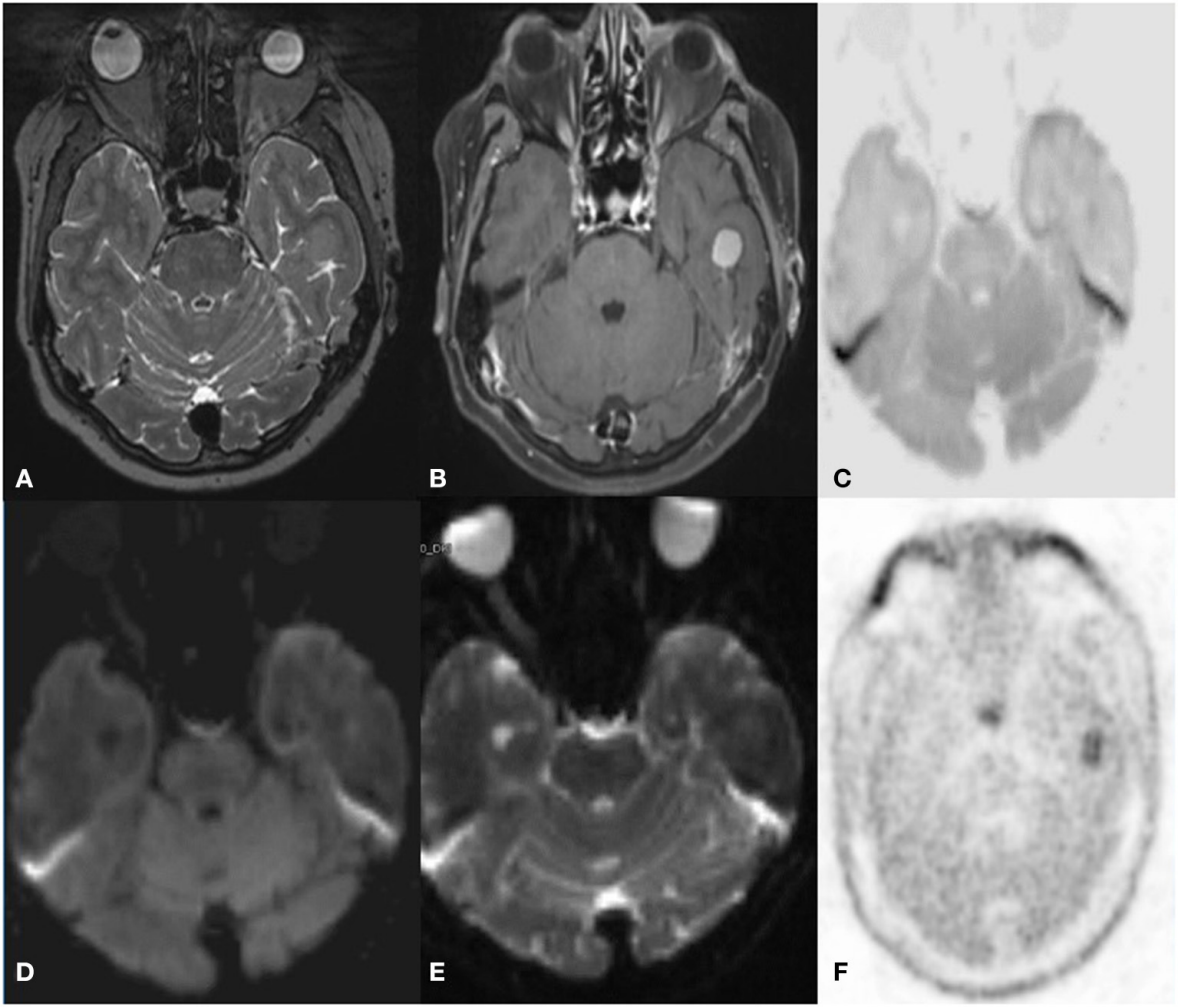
A 59 year old female with Glioma, incidentally detected a lesion in follow up MRI C-11 Methionine PET axial images T2W (A) post-contrast T1 (B) Inverted DWI (C) DWI b2000 (D) ADC (E) NAC PET (F) shows a broad-based T2 hypointense intensely enhancing in the left inferior temporal convexity. The lesion shows no DWI restriction with hypermetabolism on PET. S/O meningioma. False-positive uptake of meningioma by methionine.
